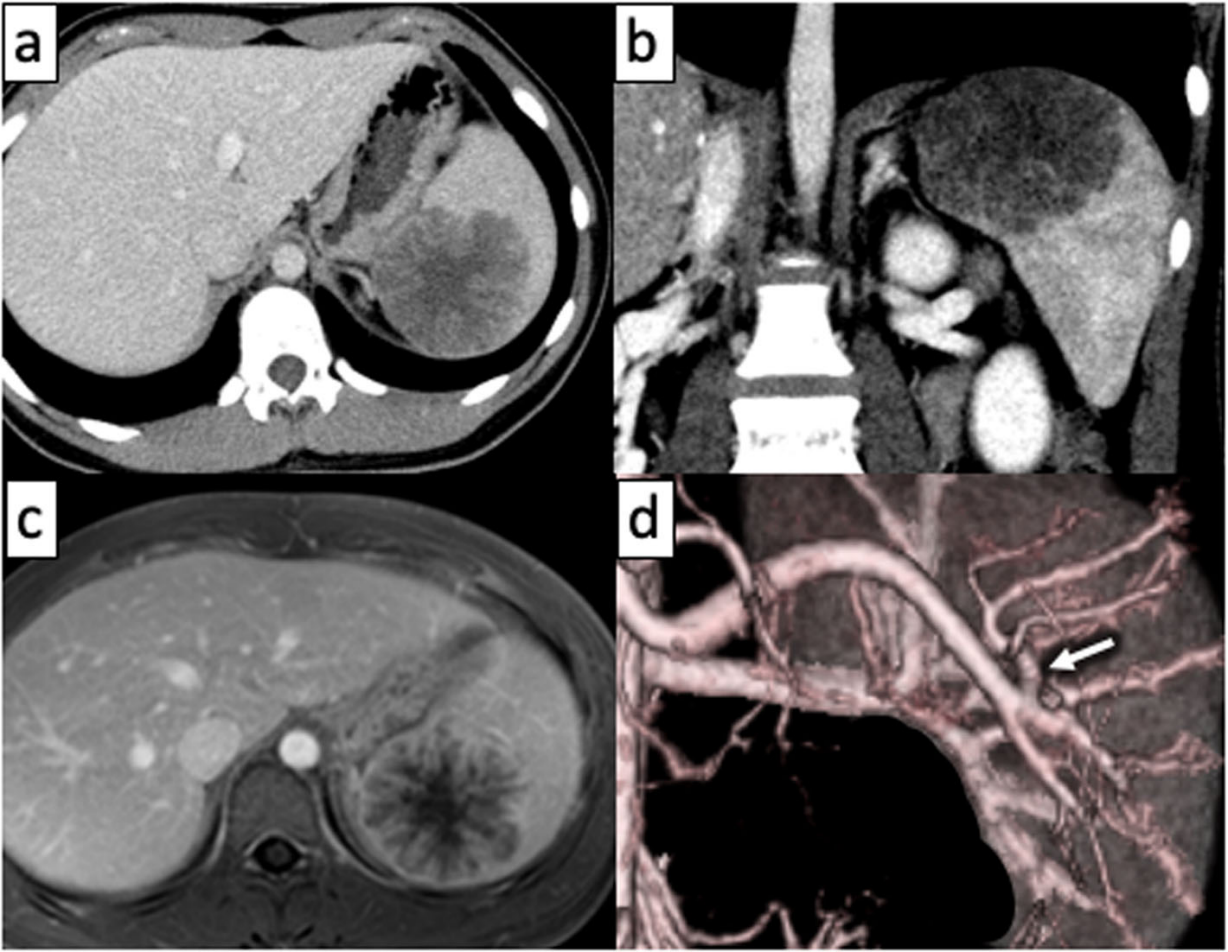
a, b A CT scan of the abdomen showing a hypodense lesion in the upper pole of the spleen. c MRI shows a mass contrasted from edge to center, the so-called spoke-wheel pattern. d Angiography showing the upper pole branch of the splenic artery (arrow)
